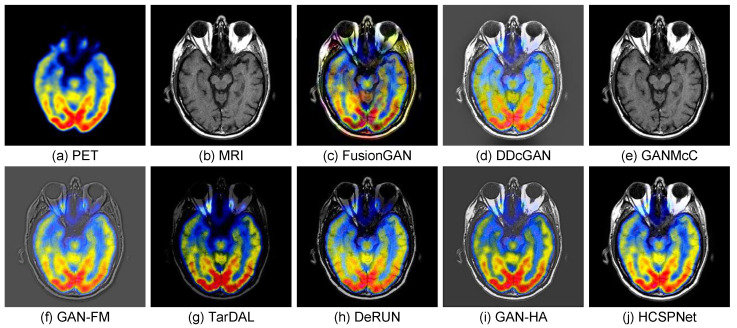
Visual comparisons of medical image fusion. (a,b) PET and MRI images. (c–j) Fused images from the compared methods.
